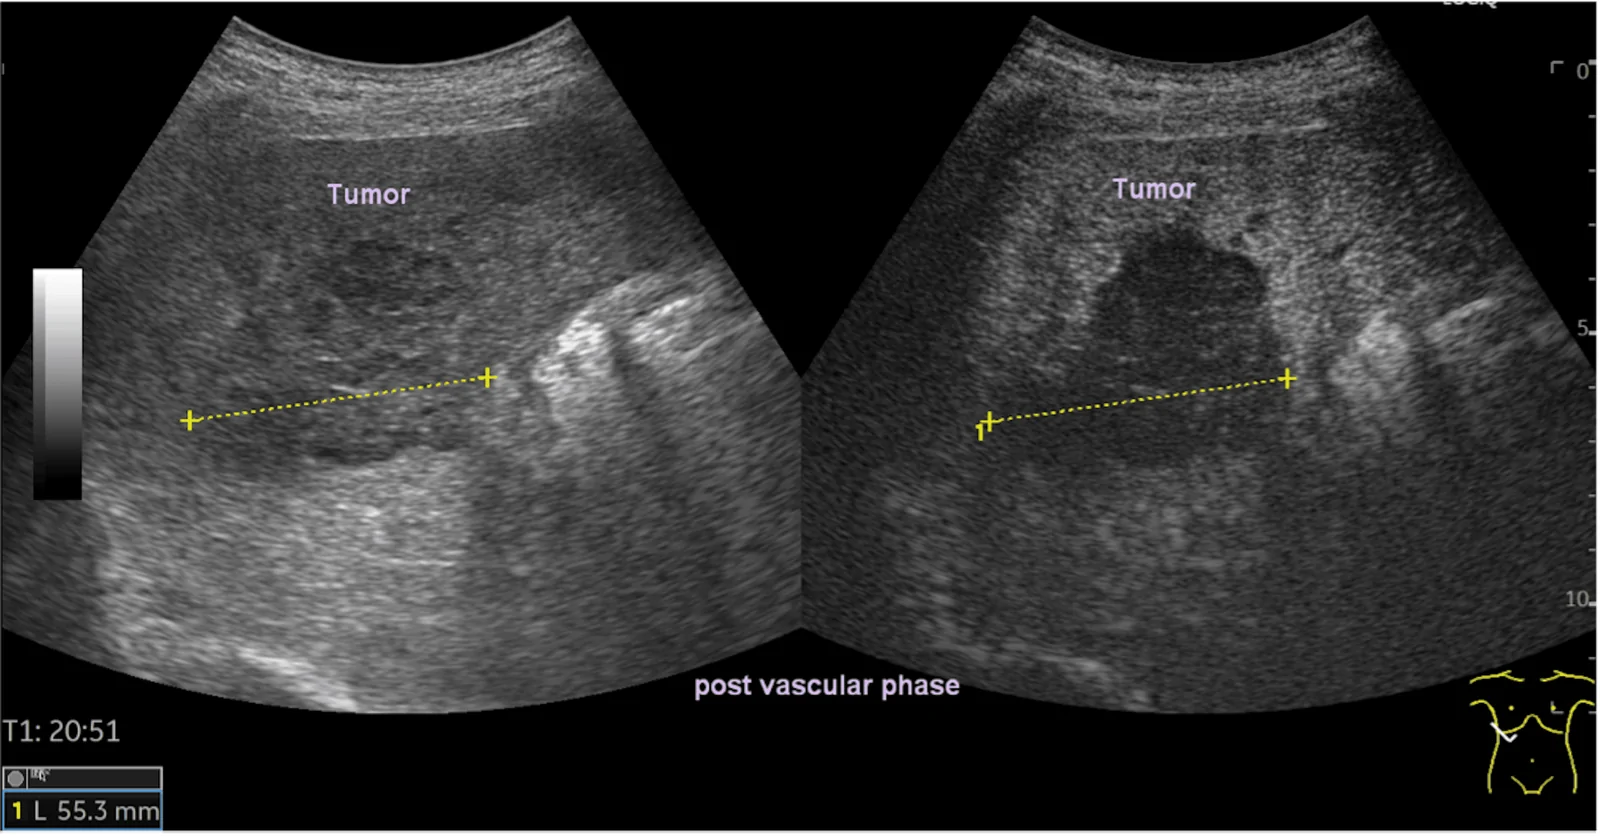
Contrast-enhanced ultrasound (CEUS) CEUS identified four metastatic lesions in the posterior segments of the right hepatic lobe. The hypoechoic tumor in segment 7 showed only faint spotty peripheral vascularity, with no discernible internal vascular structures, suggesting markedly reduced tumor viability following immunotherapy.
